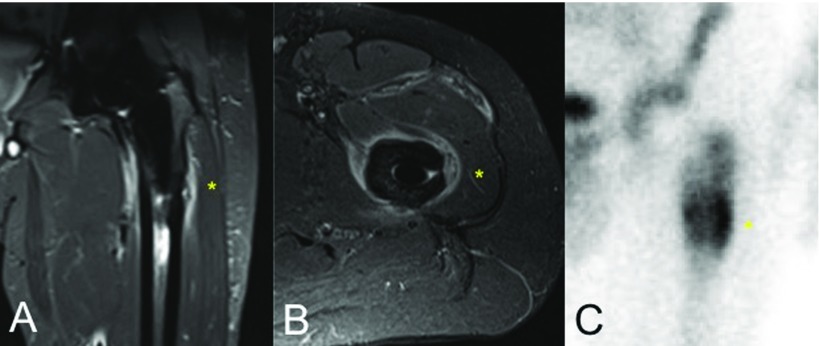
A and B. Fat-Sat magnetic resonance imaging sequences demonstrating intraosseous and periprosthetic bone oedema as well as soft-tissue inflammation at the coronal (A) and axial (B) views. C. Triple-phase bone scintigraphy showing high metaphyseal uptake surrounding femoral stem, especially at the lateral femoral cortical (*).
